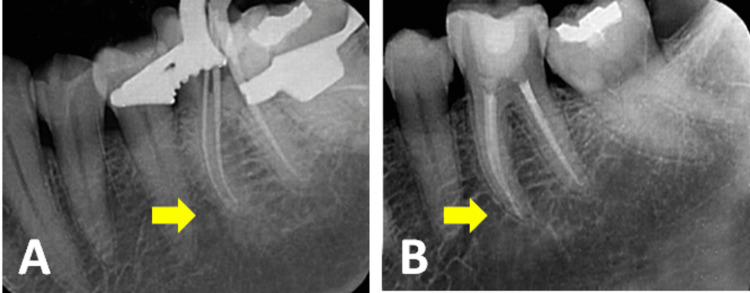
Intraoral periapical radiographs of 36 (A) Master cone verification and (B) postoperative radiograph
